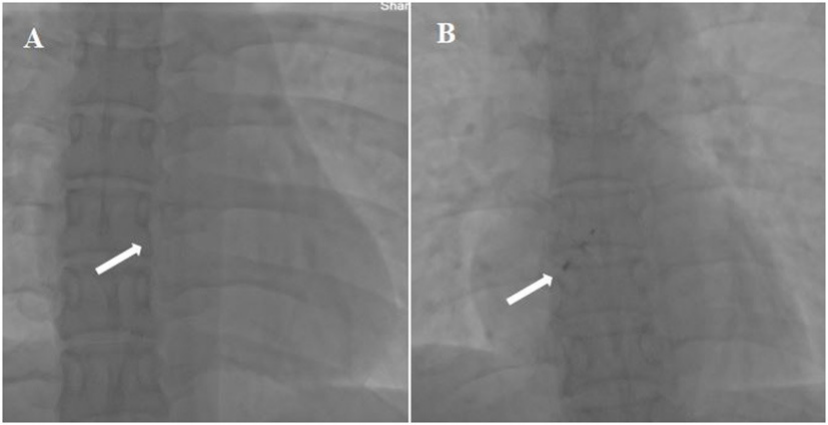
X-ray appearance of the traditional metal occluder and biodegradable occluder. (A) Non-visualization of degradable occluder on X-ray; (B) conventional metal occluder visualized under X-ray.
